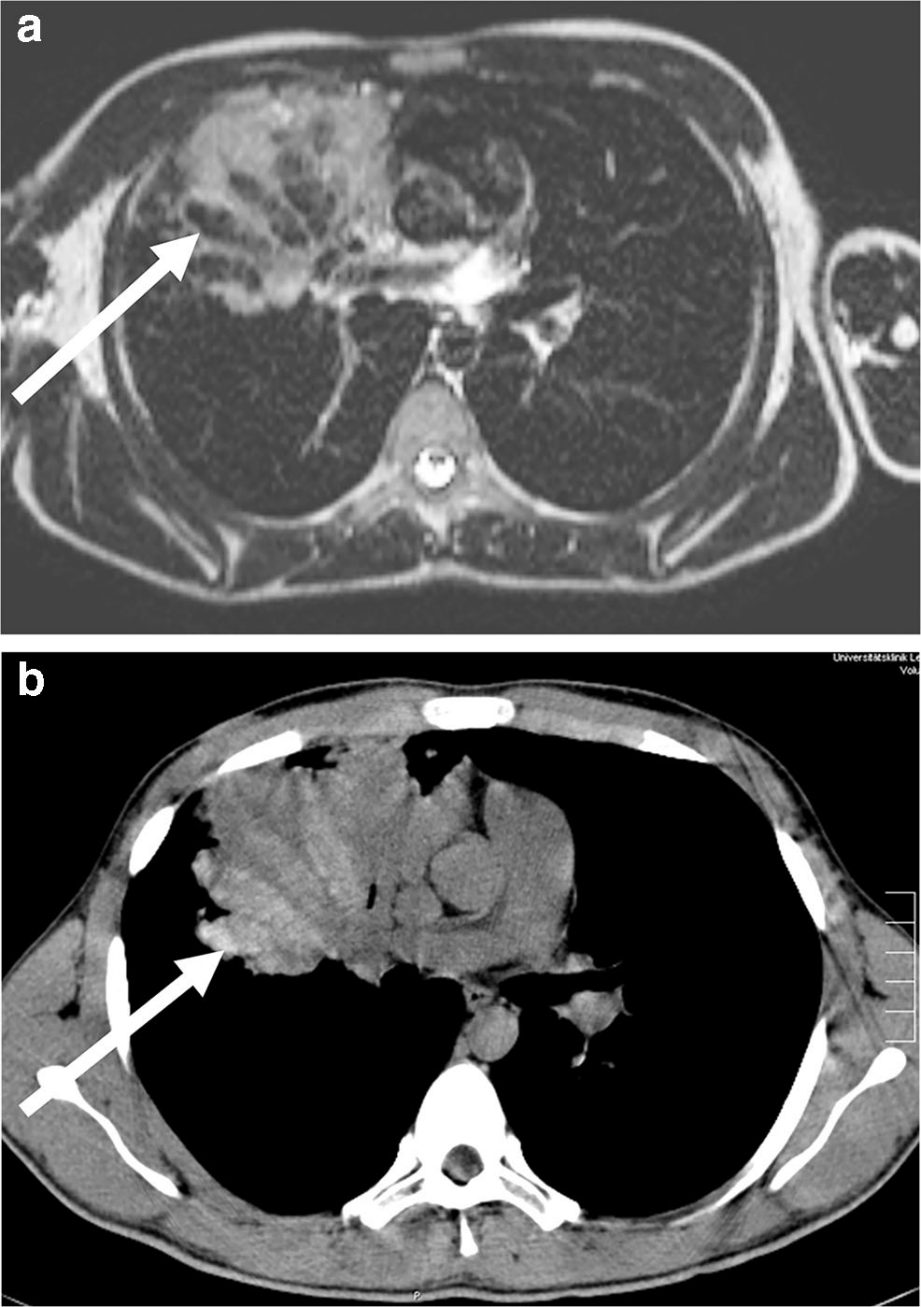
Lung MR in an 11-year-old boy with cystic fibrosis and aspergillomas within bronchiectasis. a Axial T2-weighted turbo spin-echo MR image (parameters in Table 2) shows aspergillomas in preformed cavities (*arrow*). They can be diagnosed specifically on MR by the increased iron content. They show low signal at T2 weighting. However, confusion with air in the bronchiectases is possible. b A low-dose axial CT image shows that the bronchiectases are filled with echogenic aspergillomas of high density (*arrow*)
